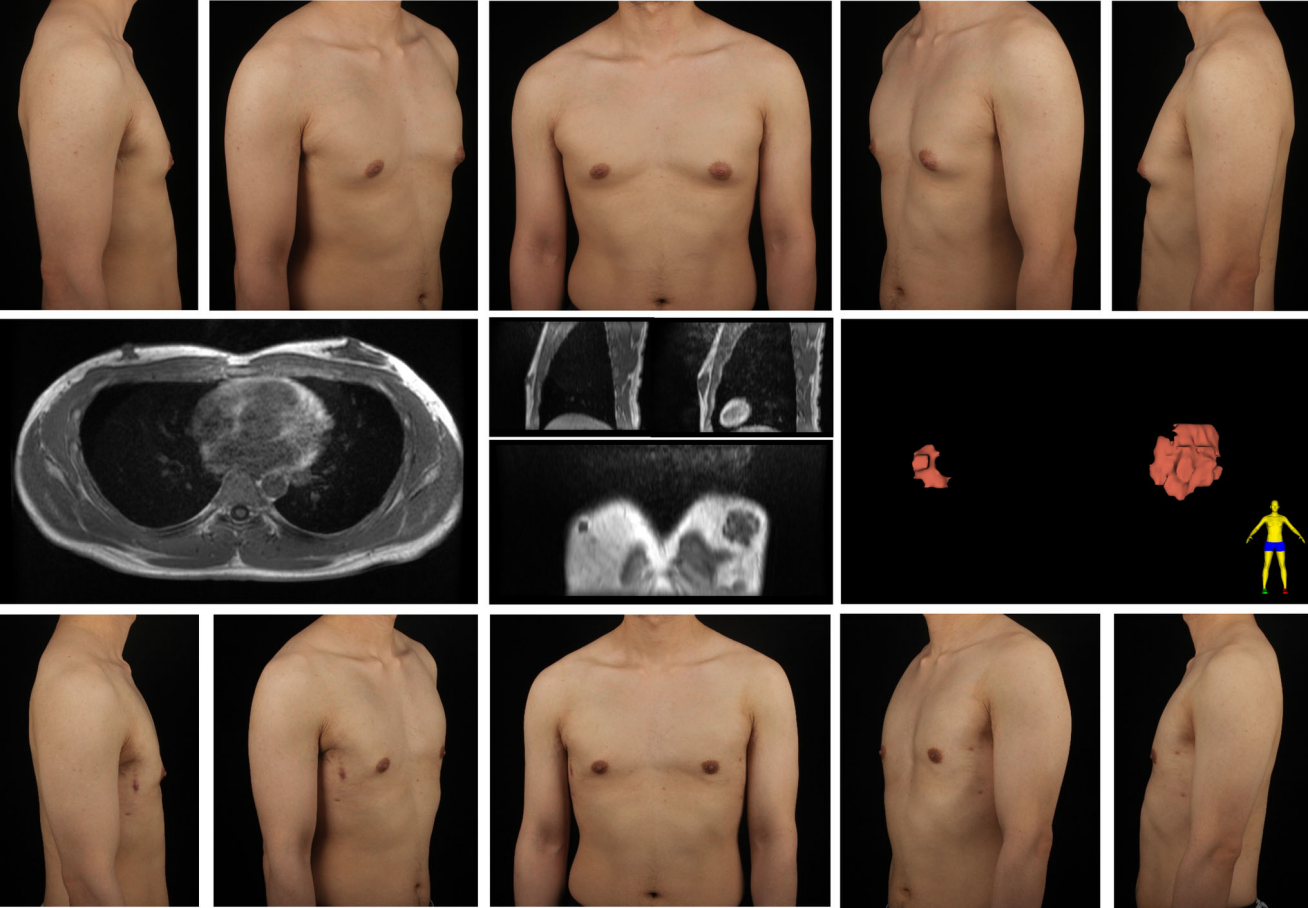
Asymmetric gynecomastia. A 30-year-old man showed grade 1/type 1C (right side) and grade 2/type 2C (left side) gynecomastia, based on chest appearance (upper) and MR images (middle). Liposuction and pull-through were performed on region 1 of right chest, while mammotome-assisted resection and liposuction were performed on region 1–2 of left chest. Chest appearance 6 months after surgery (lower)
